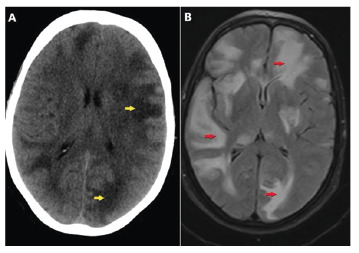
Hypodense edema in the white matter on the non-contrast axial brain tomography image (A). Hyperintense edema in the white matter of the brain on the axial T2-weighted inversion recovery scan (FLAIR) image (B).
